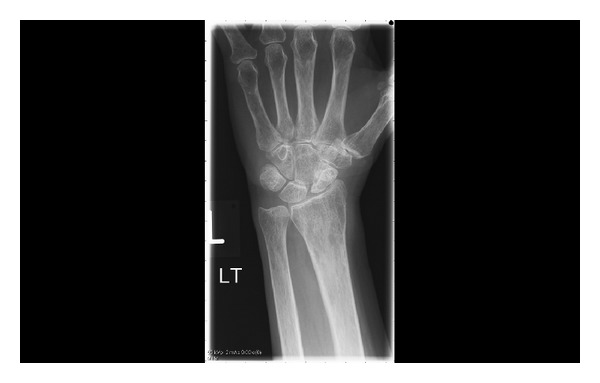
Plain AP radiograph of the carpus.
